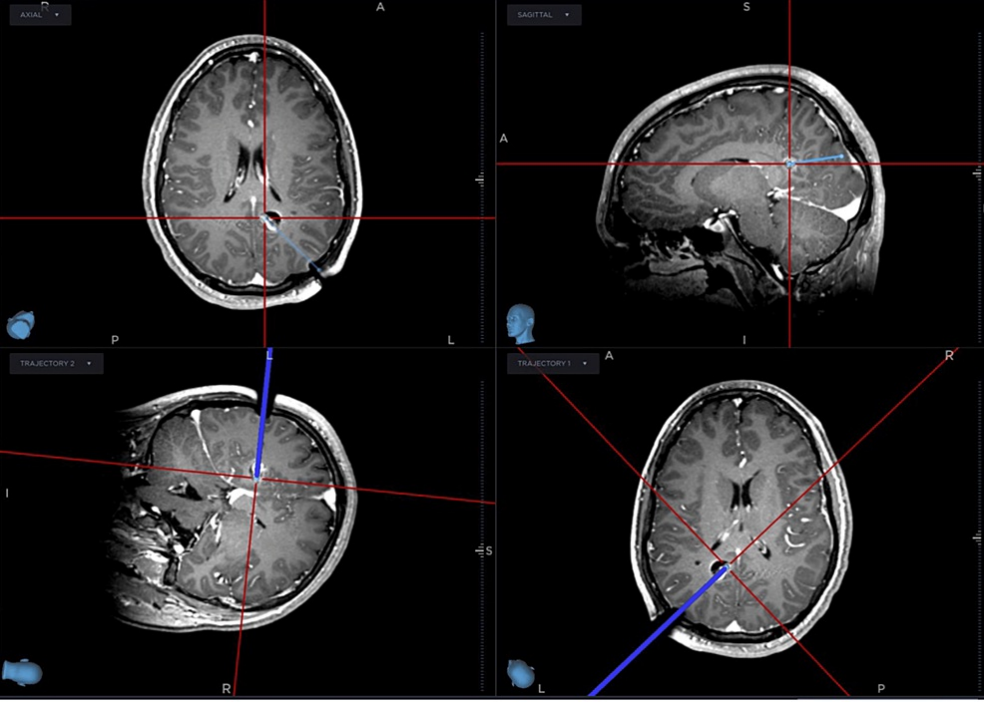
T1 Post-Contrast MRI Following Surgery From top left clockwise: Axial, sagittal, and two orthogonal trajectory views are shown extending into the ablation cavity on this T1 post-contrast MRI following surgery. The cavity measures 14.3 × 11.5 × 14.2 mm in the axial plane. MRI: magnetic resonance imaging
